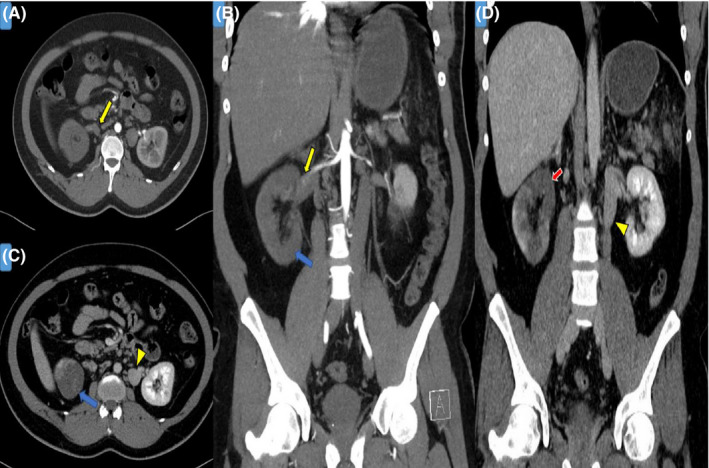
Images from Abdominal CT scan with contrast. (A and B) the arterial phase, and (C and D) the venous phase demonstrate right renal arterial filling defect (yellow arrows). The right kidney parenchyma did not fill with contrast during the arterial phase (blue arrows) comparing to the left kidney and persistent of the right upper kidney hypoattenuation during venous phase suggestive of renal infarction (red arrow)
